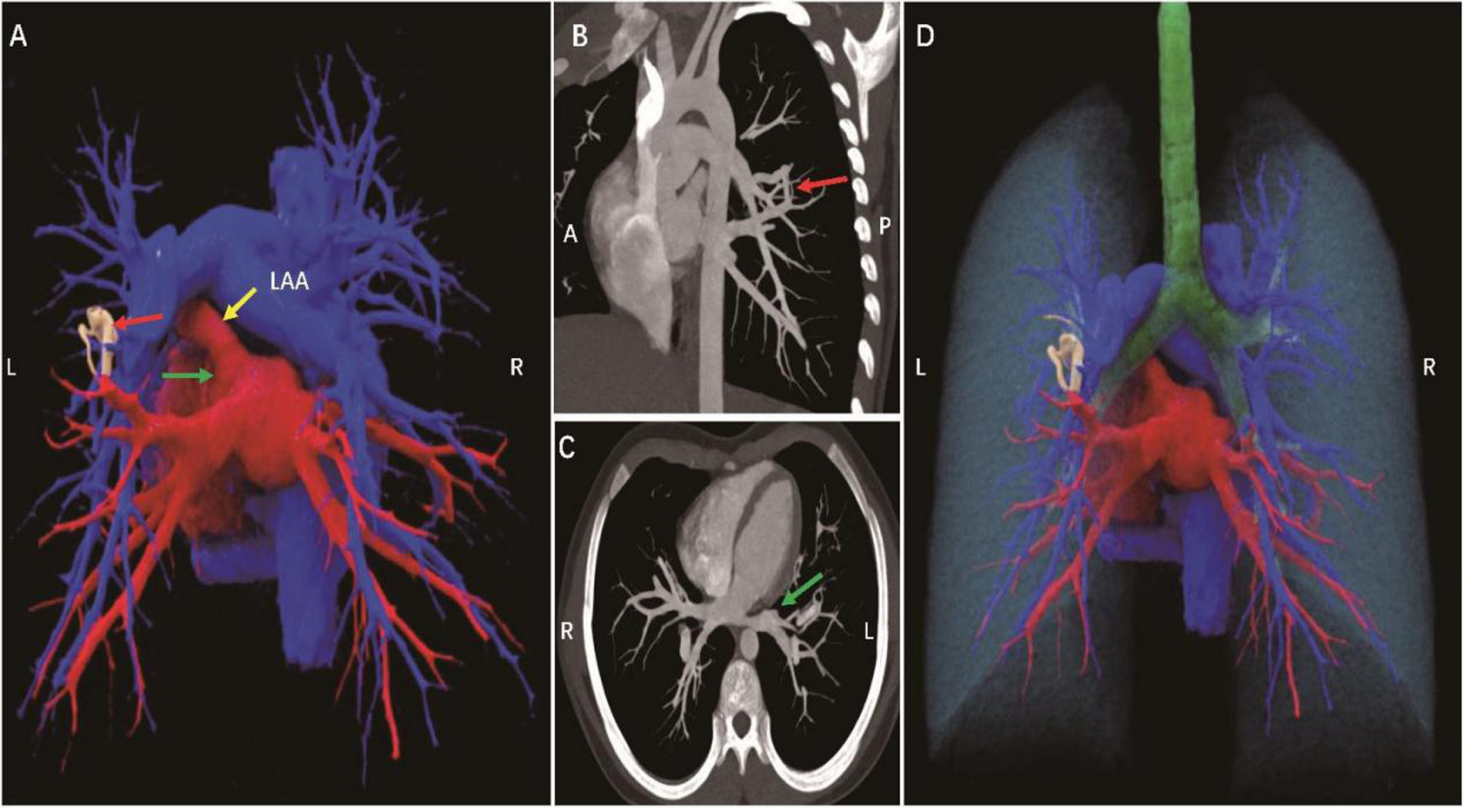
A 13-year-old female patient, pulmonary arteriovenous malformation (A). Cinematic rendering shows an abnormal vascular shadow with tortuous running and uneven thickness between the left lower pulmonary artery and the proximal left upper pulmonary vein (red arrow) (B,C). CT MIP images show that the pulmonary artery is directly connected to the vein, forming a traffic (red arrow), and the left upper pulmonary vein is not seen (green arrow). (D) Cinematic rendering clearly shows the spatial position relationship between the lung, pulmonary artery, pulmonary vein, trachea and the lesion site. A, anterior; P, posterior; L, left; R, right; LAA, left atrial appendage.
